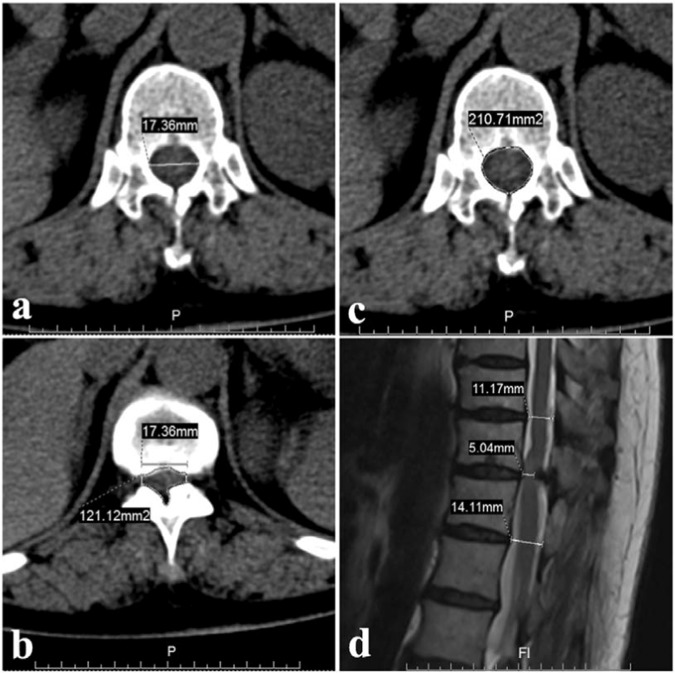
The measurement of the CSA and APD on axial CT and sagittal MRI (case 21). (a) The widest distance between two pedicles as viewed on a CT scan was measured as the transverse spinal canal diameter, equal to the transverse spinal canal diameter at the maximally compressed CT scan. (b) A vertical line extending through the endpoints of the transverse diameter determined the boundary of the spinal canal and was used to measure the compressed CSA. (c) The normal CSA was measured on the pedicle section of the same vertebrae. (d) The APD was measured at the compressed level as well as at two normal levels above and below the compressed level.
